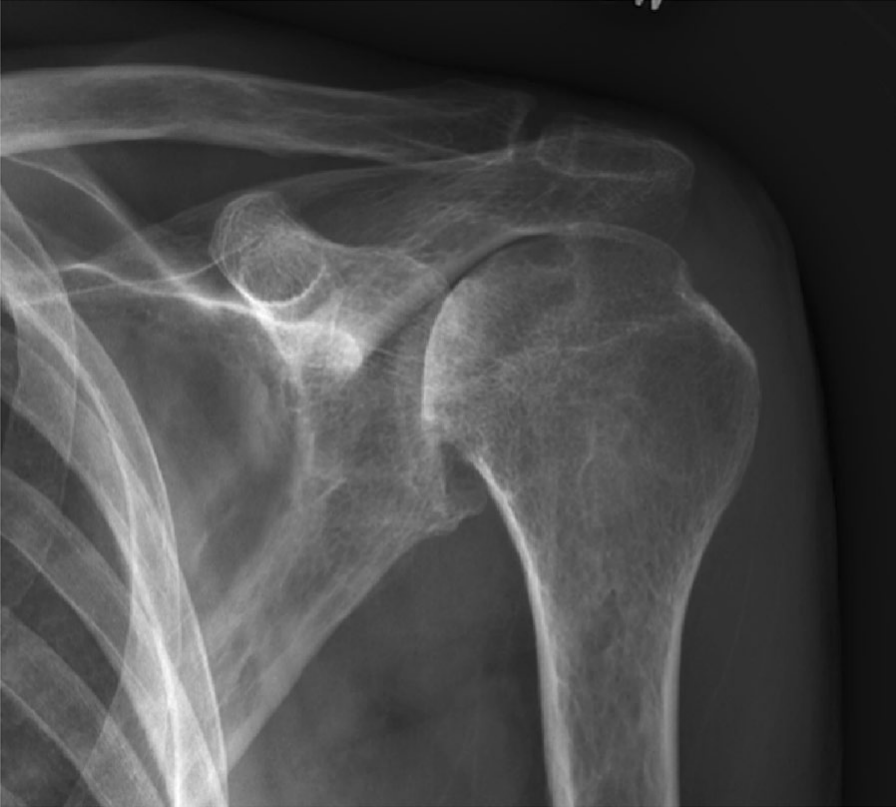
Radiograph of the left shoulder revealing marked glenohumeral joint space narrowing, small humeral head osteophytes, subchondral sclerosis, and subchondral cysts
